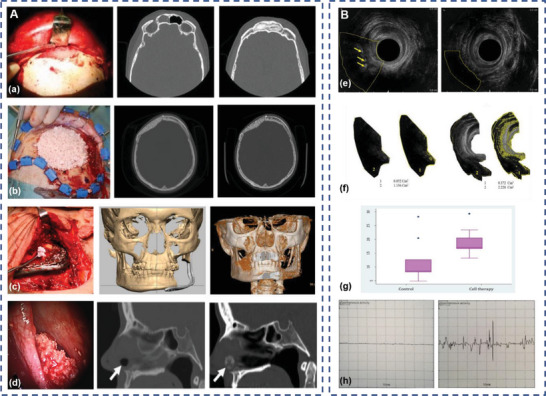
Clinical application of adipose‐derived stem cells (ADSCs) for cranio‐maxillofacial hard‐tissue regeneration and external anal sphincter injury treatment. A) Intraoperative photograph and computed tomography (CT) of frontal sinus regeneration (a), cranial repair (b), mandibular reconstruction (c), and nasal septal repair (d) using ADSCs. Reproduced with permission.^[^
